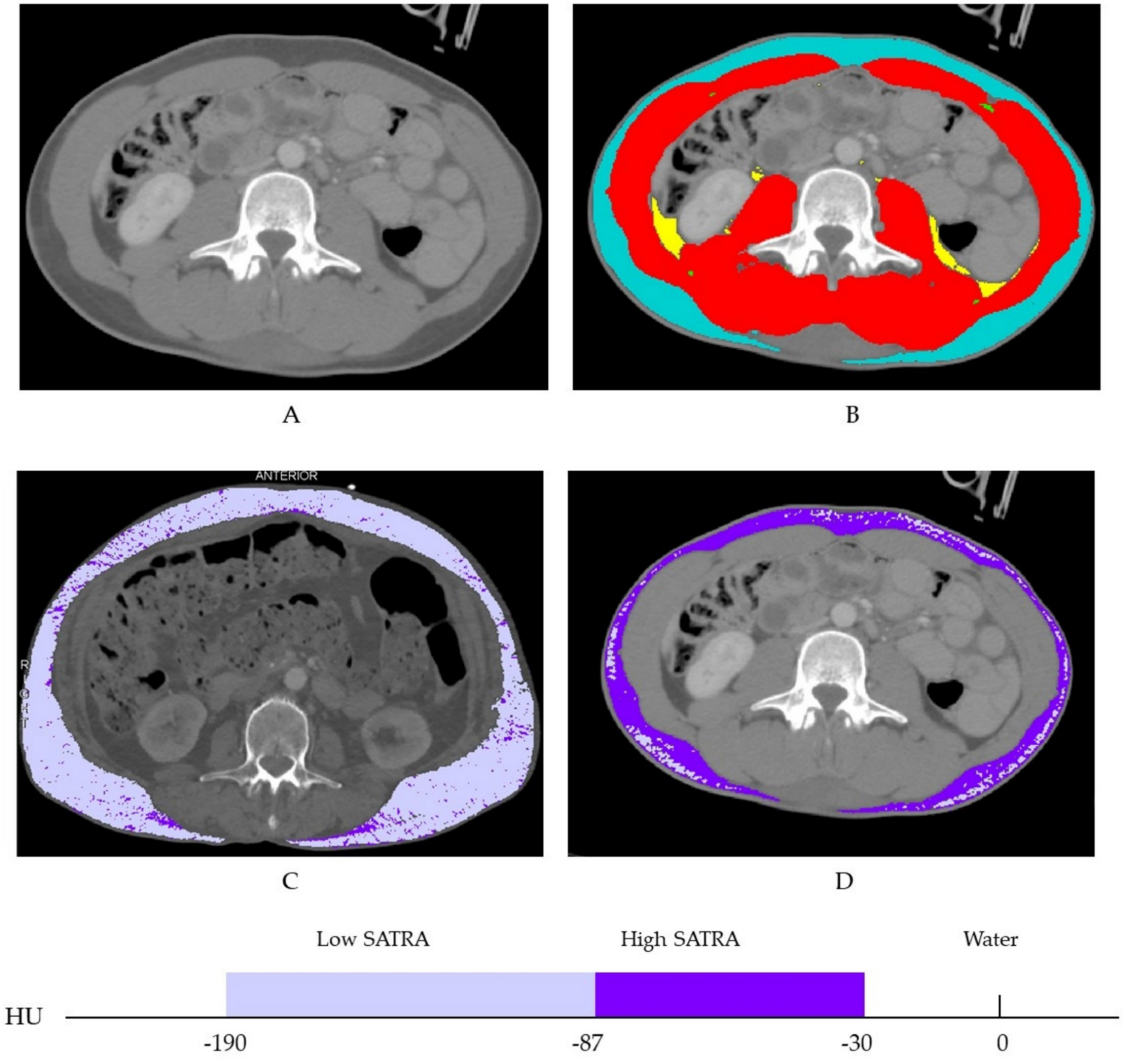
Body composition analysis at the third lumbar vertebra with SATRA characteristics of two patients applied for SATRA assessment. (A) Original CT slice on third lumbar level. (B) Segmented skeletal muscle, visceral adipose tissue and subcutaneous adipose tissue are delineated in red, yellow and blue, respectively. (C,D) Comparison of two patients with (C) a high low > − 87.54 SATRA and (D) with a low high <−87.54 SATRA (darker shade of purple, indicative of high triglyceride concentration).
